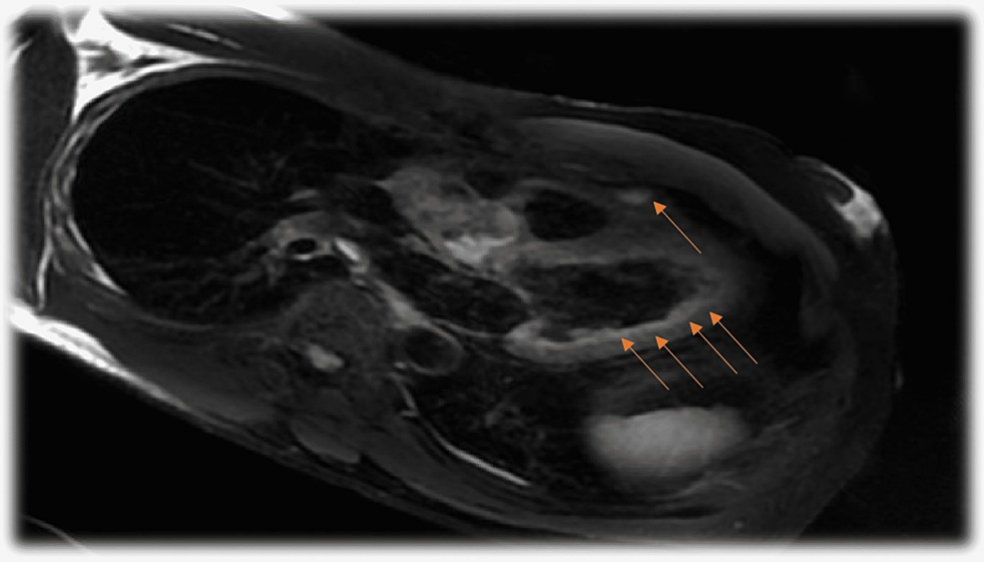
Cardiac MRI showing multifocal myocardial late gadolinium enhancement (arrows) consistent with myocarditis
